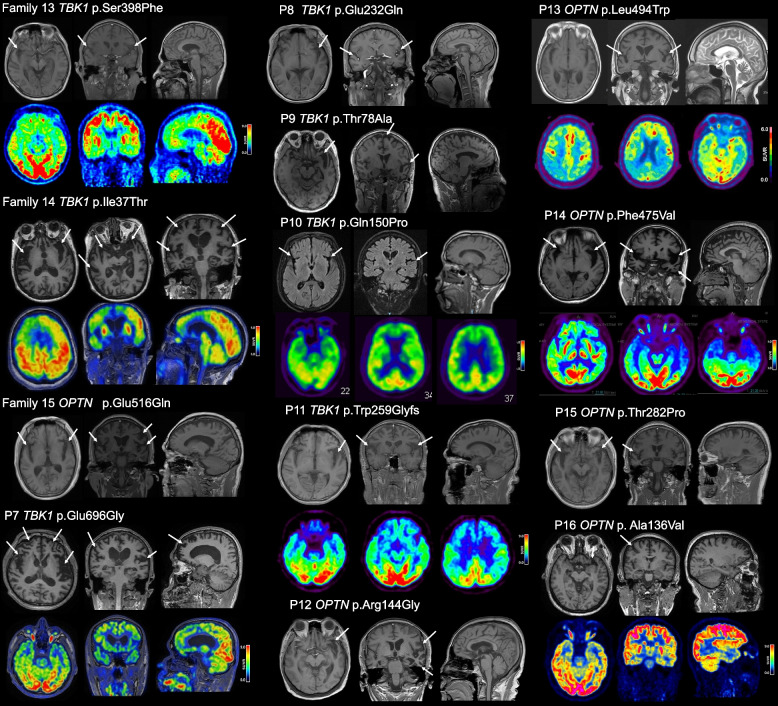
Neuroimaging studies of the FTD patients with *TBK1* and *OPTN* variants. The upper lane shows the cranial MRI. The atrophic changes in frontotemporal regions are indicated by white arrows. The lower lane shows the ^18^F-FDG-PET images. The color bar of the ^18^F-FDG-PET images indicates the corresponding regional standardized uptake value ratios (SUVR)
